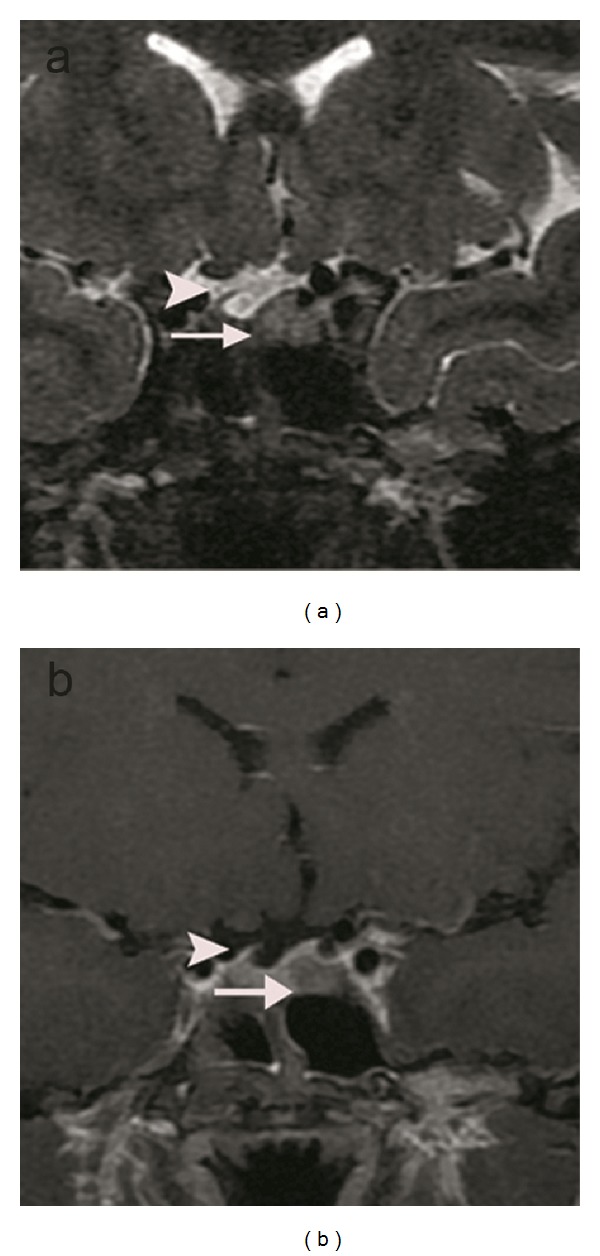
T2-weighted coronal image (a) shows a suspicious hyperintense lesion on the left side of the gland. T1-weighted coronal image enhanced with IV-GBCM (b) shows the lesion as a hyperintense lesion on the left side of the gland. Infindibulary deviation (arrow heads) and diffuse enlargement of the pituitary gland can be observed on both images.
